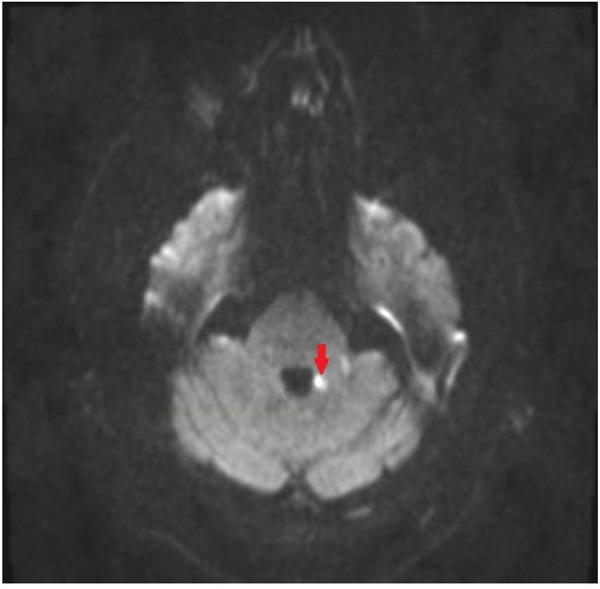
Magnetic resonance imaging (MRI) of brain of patient. Diffusion weighted image showing restricted diffusion in the medial aspect of the left middle cerebellar peduncle.
